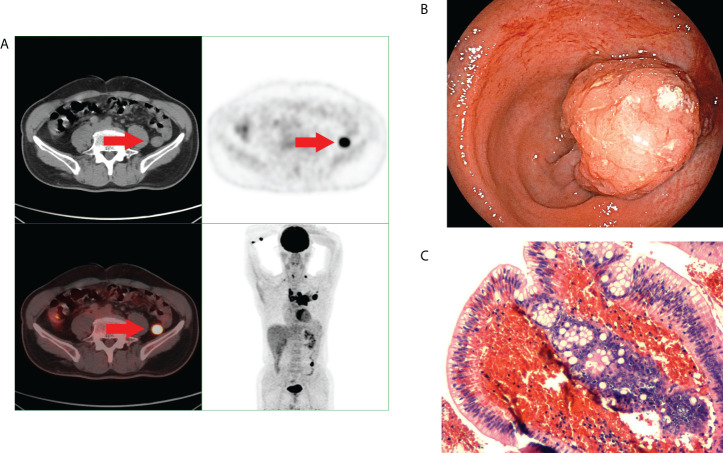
A middle-aged man underwent 18F-FDG PET/CT for left pulmonary nodules. Incidental focal uptake of 18F-FDG was found in the rectum. The patient underwent colonoscopy and pathology examination. (A) Abnormal uptake of 18F-FDG PET/CT in the rectum. (B) shows a colonoscopic pedicled polyp with a diameter of approximately 2.0 cm. (C) shows the pathological type of villous tubular adenoma.
